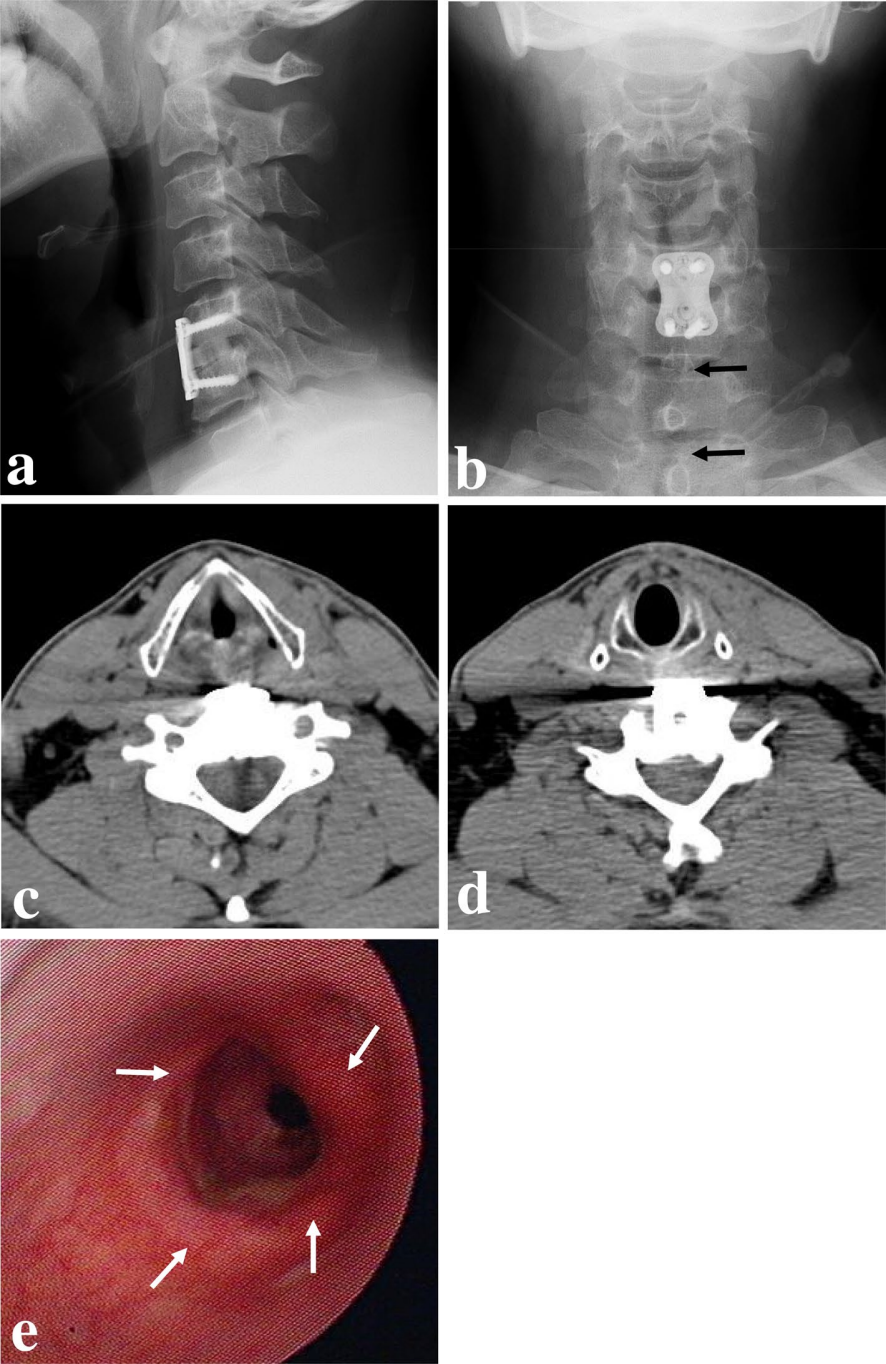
One month after hematoma evacuation surgery, follow-up lateral (a) and anteroposterior (b) radiographs showed normalization of the previous anterior soft tissue swelling and tracheal deviation (dark arrows). Follow-up neck-enhanced computed tomography scans (c and d) showing normalization of the previous anterior soft tissue swelling and tracheal deviation. Follow-up laryngoscopy (e) showed tracheal stenosis and stricture (white arrows)
